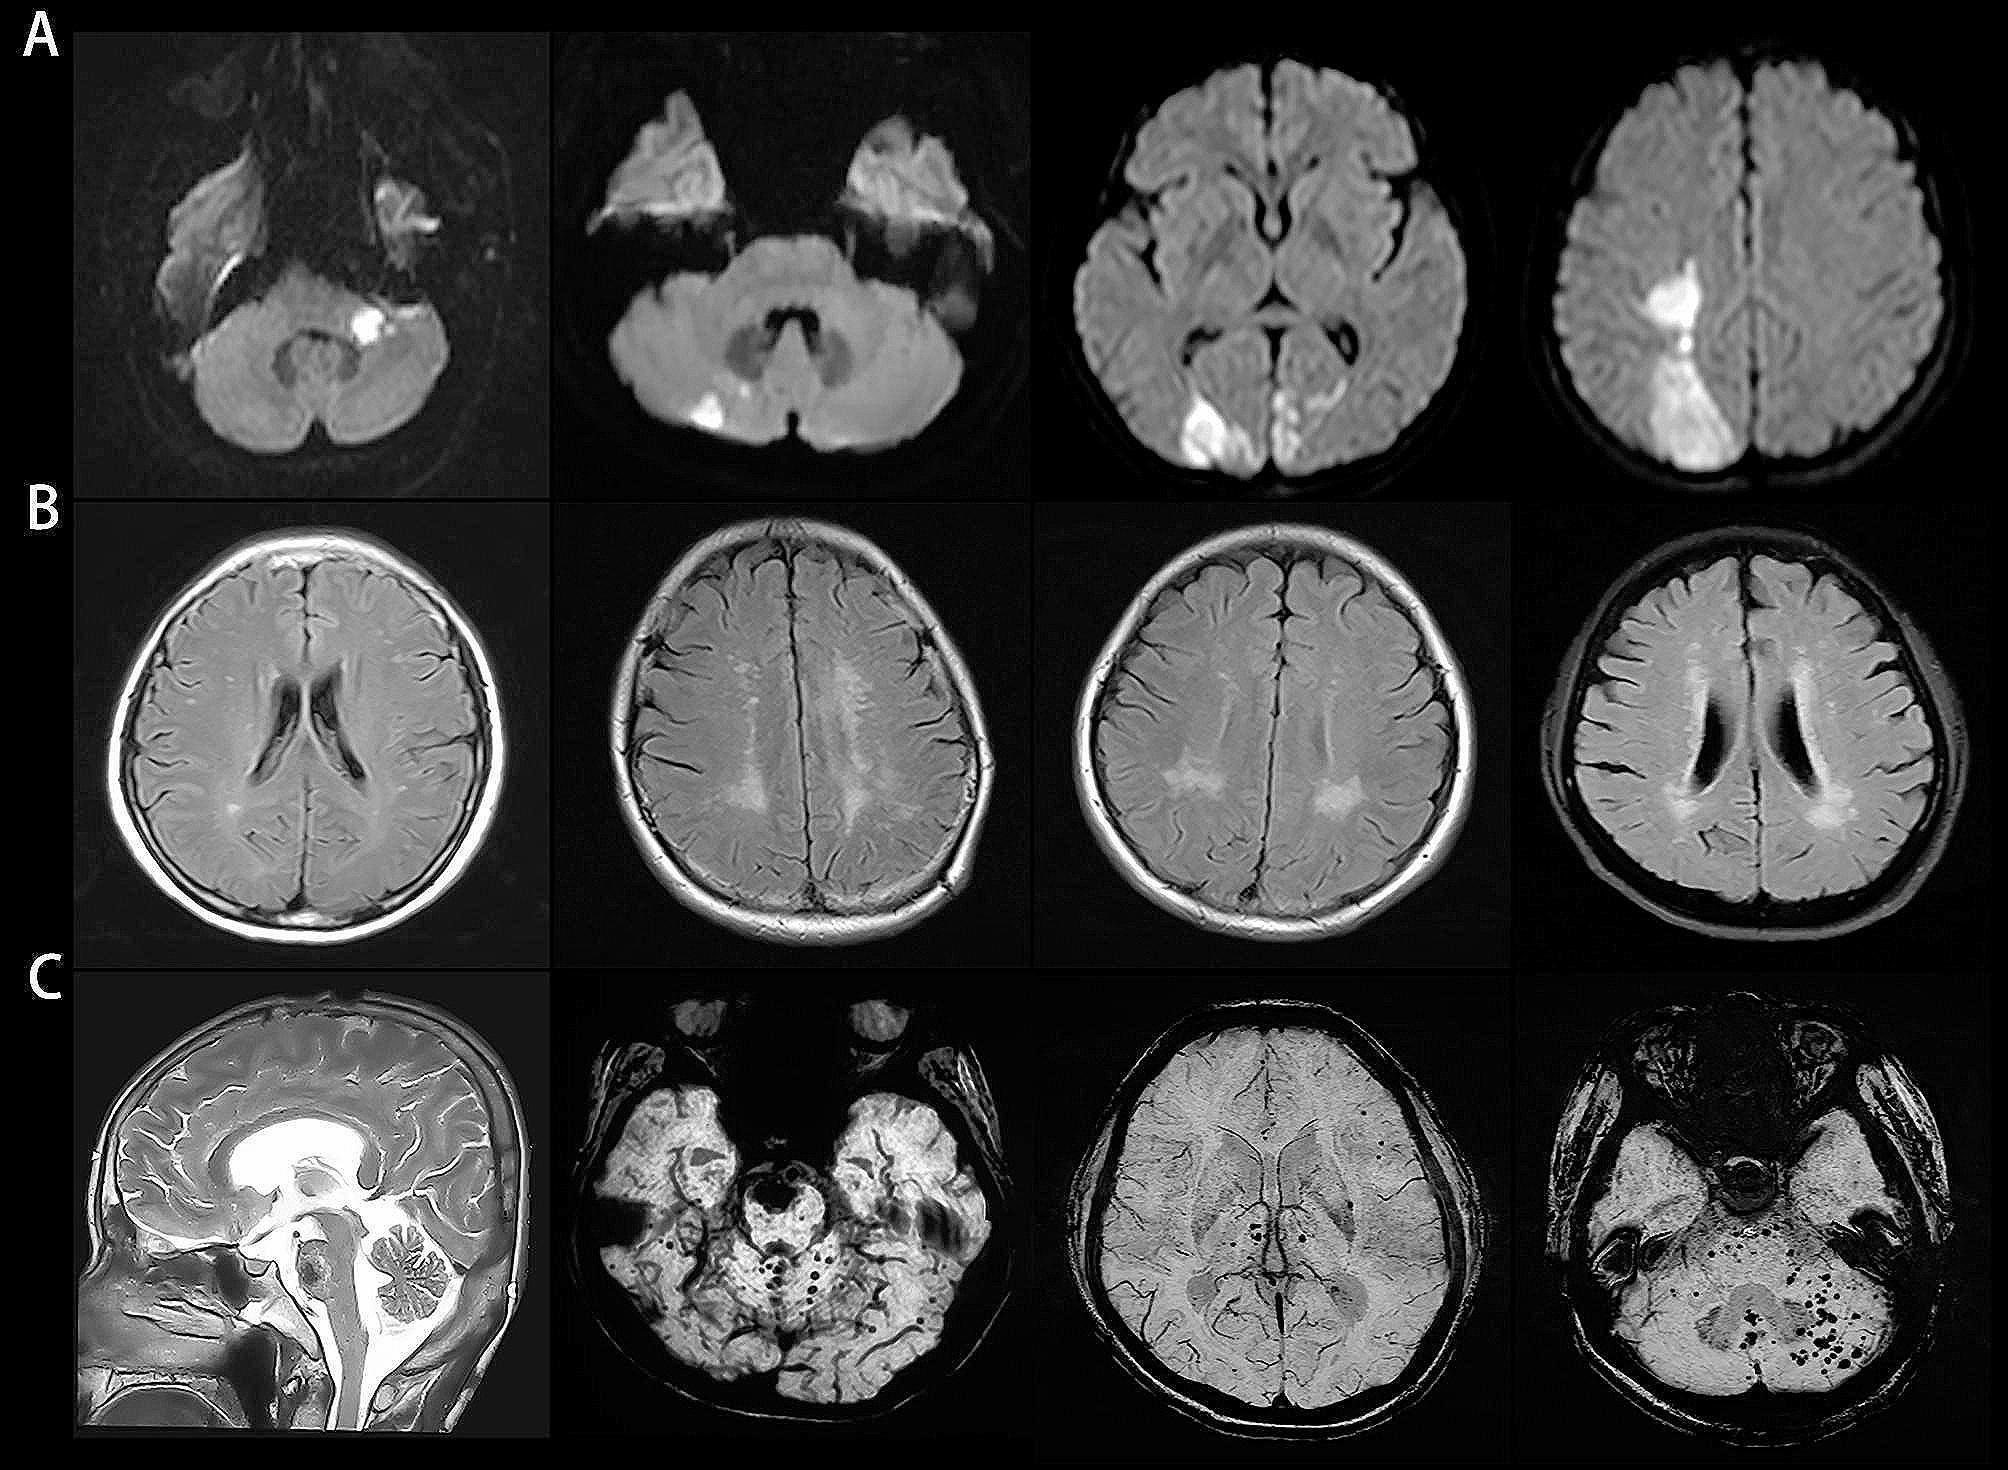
Brain parenchyma abnormalities in adult LOPD patients. (A) The infarct lesions were located at the brachium pontis, cerebellum and cerebral hemisphere on diffuse weighted imaging. (B) T2-fluid-attenuated inversion-recovery images showed white matter hyperintensities. (C) Hematoma in the brainstem and microbleeds in the brainstem, cerebellum, cerebral hemisphere and thalamus were detected via T2-weighted imaging and susceptibility-weighted imaging
